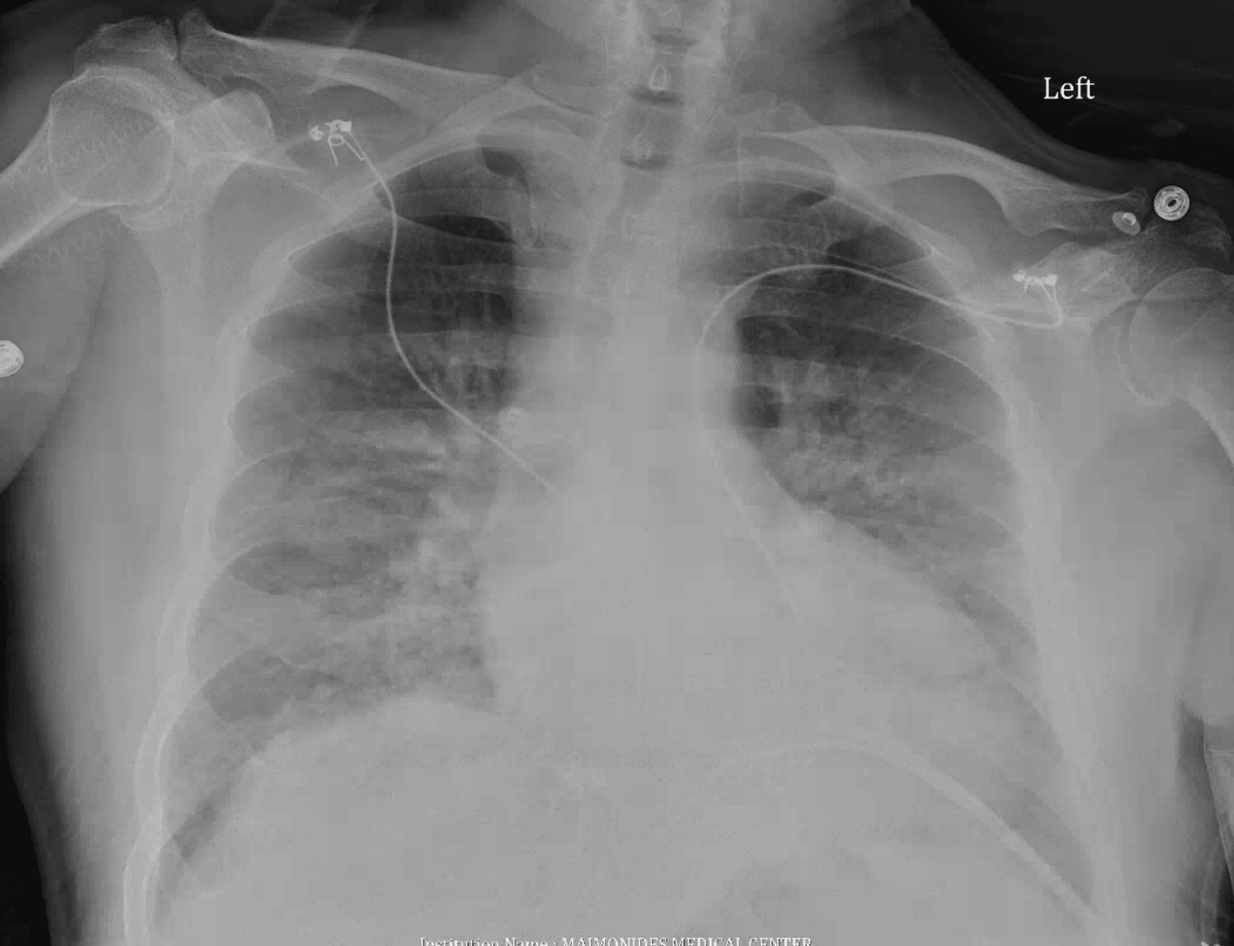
Chest X-ray Anterioposterior chest X-ray suggestive of pulmonary congestion/edema.
